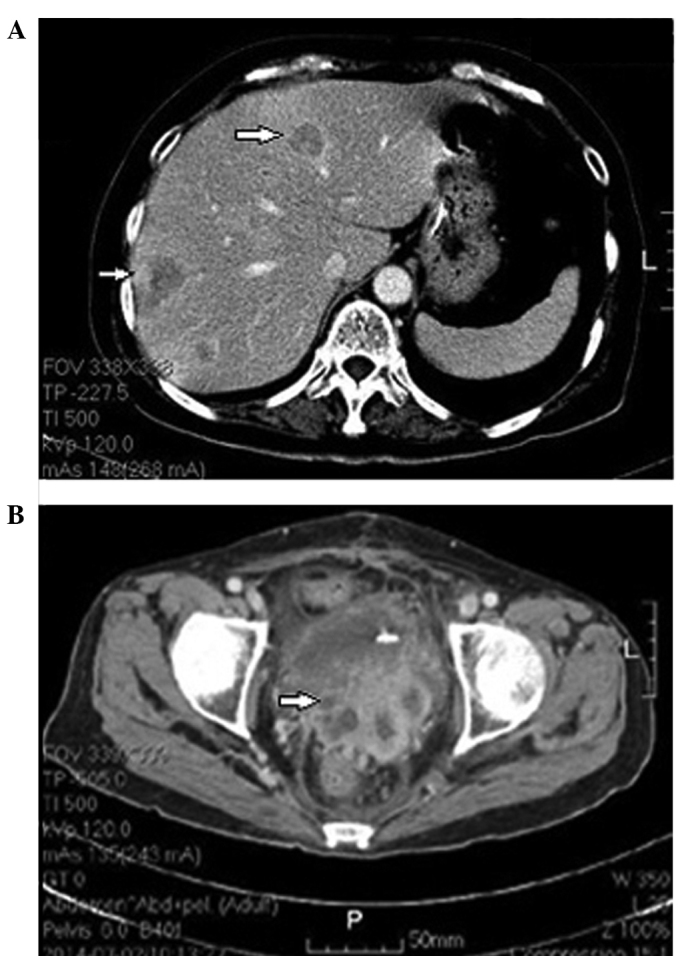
Computed tomography scans revealing multiple metastases in the (A) liver and (B) pelvic recurrence.
